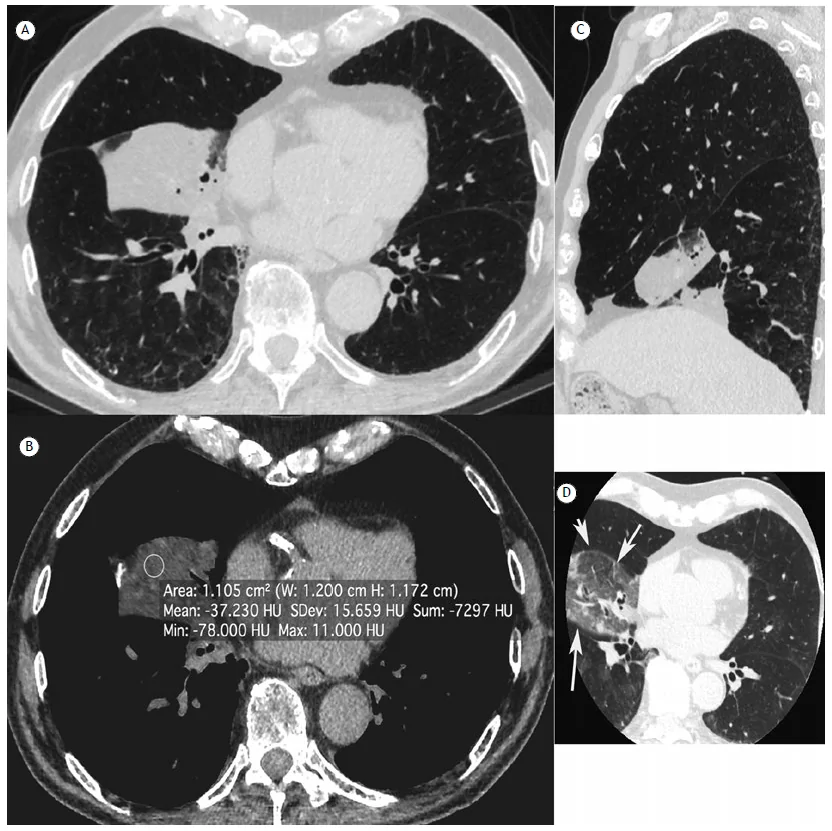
Chest CT images with axial (A) and sagittal (B) reconstruction showing consolidations in the middle lobe and the anterior basal segment of the right lower lobe. In (C), the middle lobe consolidation demonstrates negative attenuation (−37 Hounsfield units). In (D), an axial CT examination performed 2 years previously for coronary evaluation shows rounded ground-glass opacity (arrows) at the site of the subsequent middle lobe consolidation.
